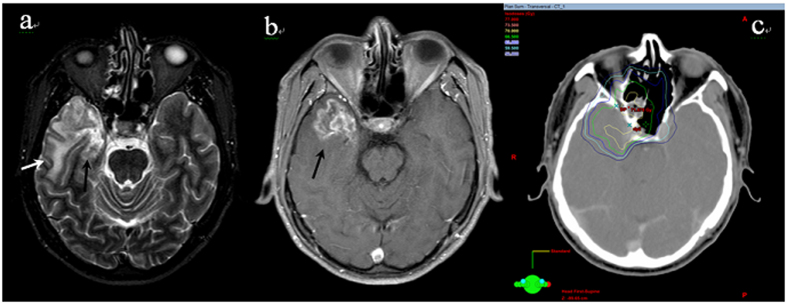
An example of TLI lesion showing (a) homogeneous high signal area in the white matter on T2-weighted images (white arrow) and the medial necrosis (black arrow) (b) contrast-enhanced lesion on postcontrast T1-weighted MR images with internal necrosis (black arrow) (c) corresponding isodose lines.
